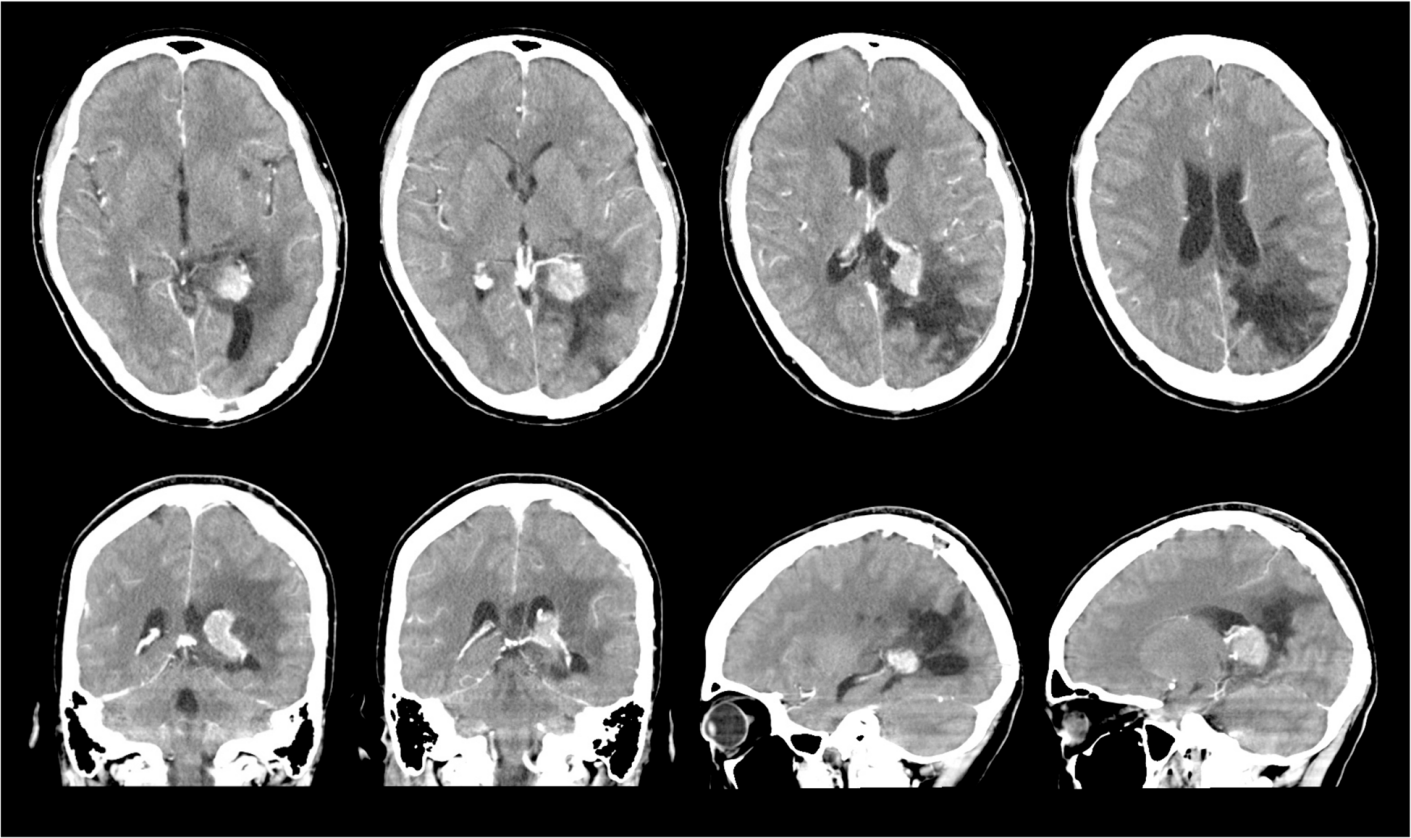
Postcontrast CT scan on day 38 after first surgery showing an enhanced small residual tumor in the trigone of the left lateral ventricle and enlarged feeding artery
